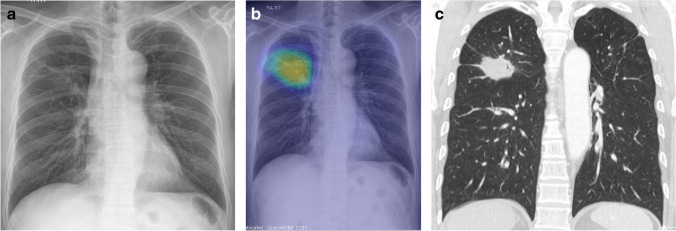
A 56-year-old man with adenocarcinoma of the right upper lobe. A chest radiograph shows a faint nodular opacity in the right upper lung zone. a The AI solution correctly detected the lesion with a probability value of 63%. b Chest CT with contrast enhancement demonstrated a spiculated nodule in the right upper lobe. c Among the 12 observers, two observers, including one pulmonologist and one radiology resident, could detect the lesion without AI assistance (unaided reading). In addition, two observers, one thoracic radiologist, and one pulmonologist marked a false-positive lesion in unaided reading. With the use of an AI solution, observers could detect the lesions. The false-positive lesion marked on unaided reading was withdrawn by two observers in AI-assisted reading. Regarding visual certainty for the lesion, three observers, including two thoracic radiologists and one pulmonologist, rated a higher score in AI-assisted reading than in unaided reading
